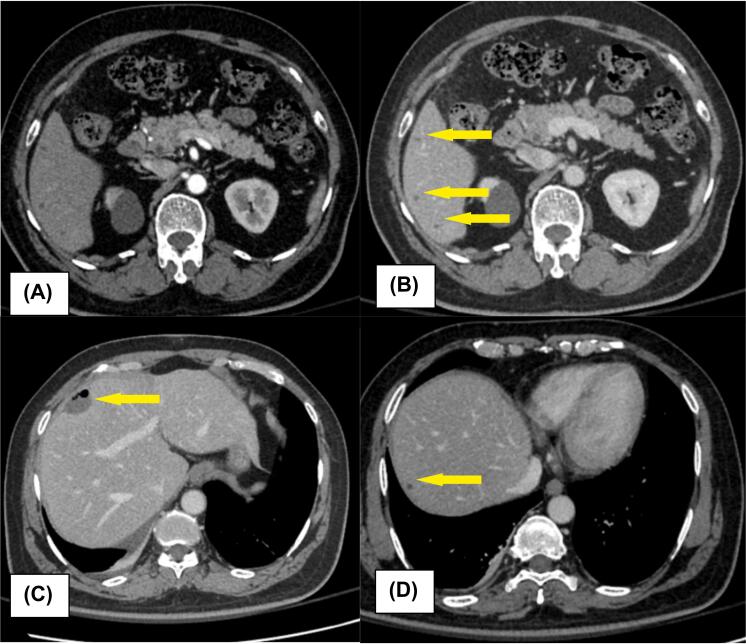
CECT liver scan findings (A) Axial sections of arterial phase, (B) Axial sections of CT (Venous phase) showing multiple bile duct adenomas(BDA) in segment VI, (C) Laparoscopic wedge biopsy site with post-surgical changes, (D) Segment VIII BDA.
